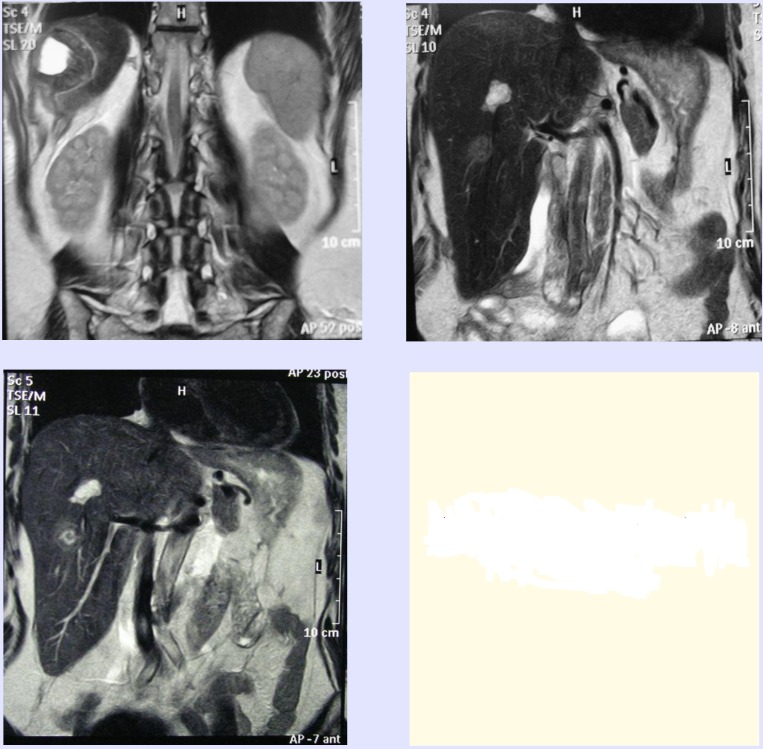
Postoperative MRI after RFAR with afterwards RFA of metastases and liver hemangioma
